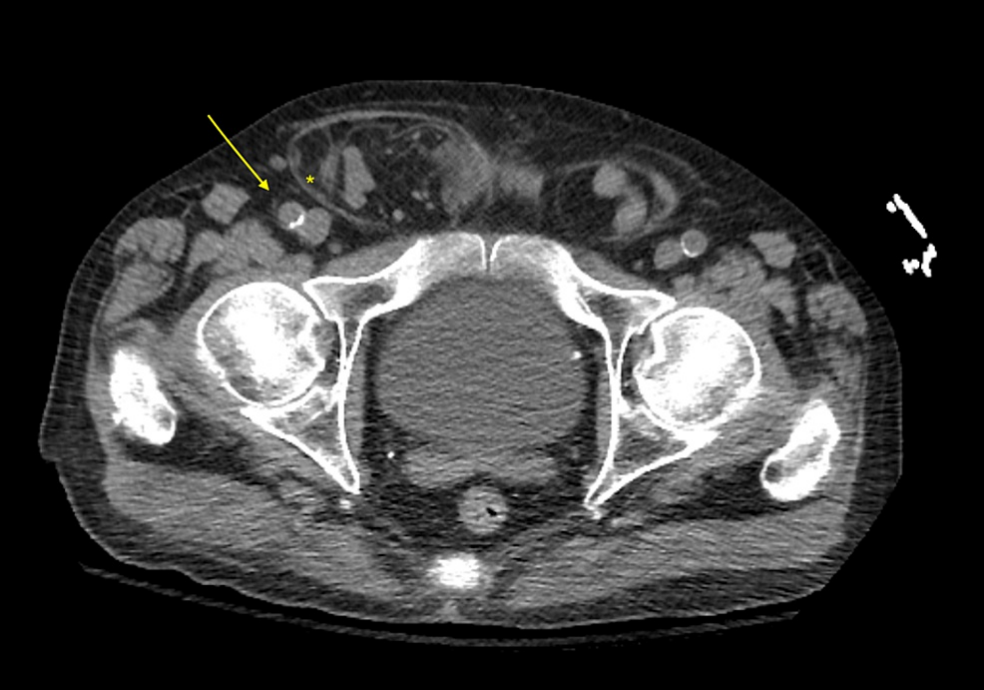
CT scan demonstrating the small bowel extending all the way into the right hemiscrotum through the right inguinal canal. CT, computed tomography
